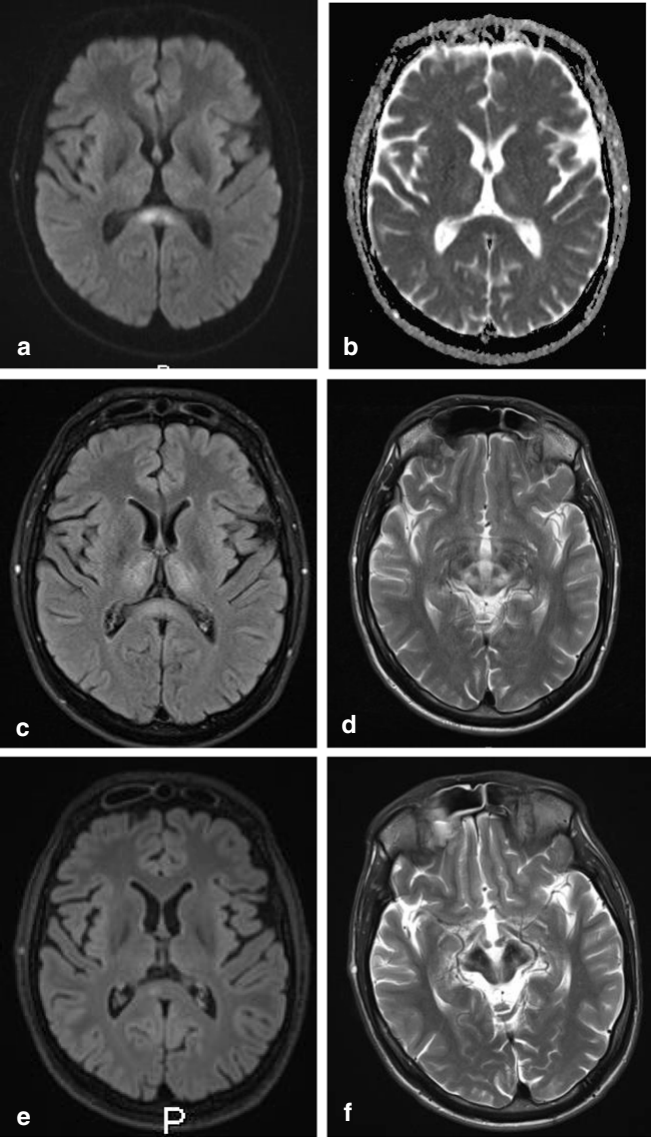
Thirty-year-old man. Axial DWI (a) showing a central area of high signal in the splenium, without low signal on ADC (b) and comparable high signal on FLAIR (c), compatible with T2 shine through effect and white matter edema. Axial T2 (d) shows a typical giant panda sign at the level of the mesencephalon-pons. On follow-up 2 years later, the DWI (e) shows a small residual splenial lesion on the right, and axial T2 (f) at the level of the mesencephalon shows atrophy and increase in low signal in the substantia nigra and nucleus ruber
